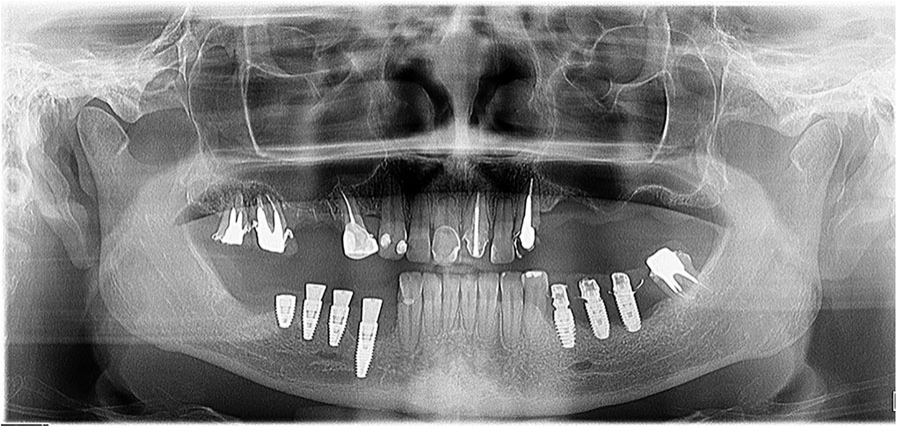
Panoramic radiograph after dental extractions with subsequent dental implants
